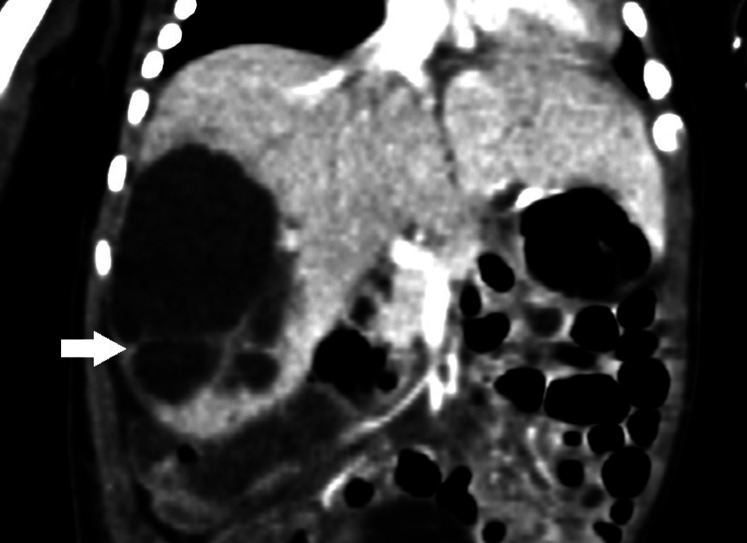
Computed tomography (CT) scans of the abdomen. CT scans showed a circular cystic mass in the right lobe of the liver, with multiple linear partitions and clear boundaries. On contrast enhanced CT scans, the capsule and septum enhanced, but no enhancement was found inside the tumor (arrow).
